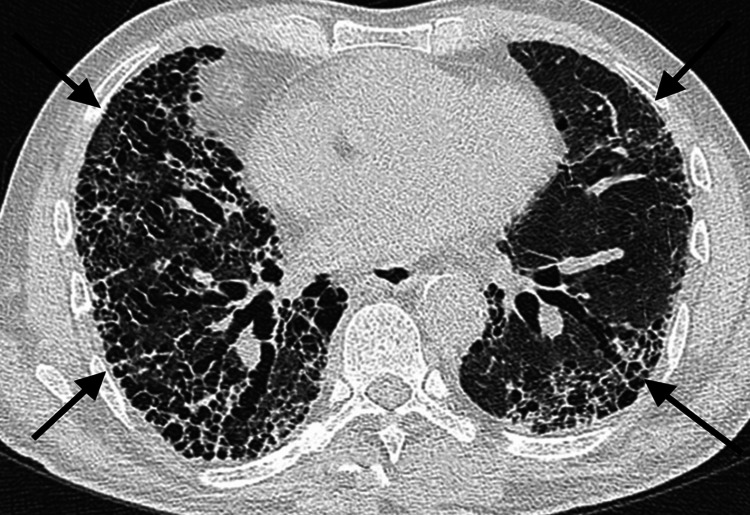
Reticular opacities with honeycombing patterns in an HRCT slice, as marked by arrows.
